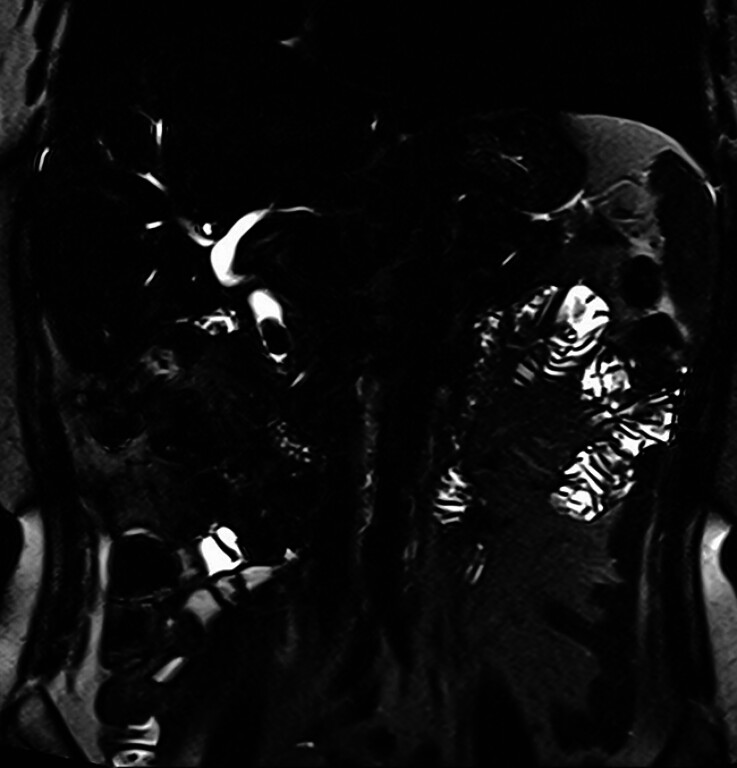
Magnetic resonance imaging revealed an 18-mm stone in the common bile duct.
